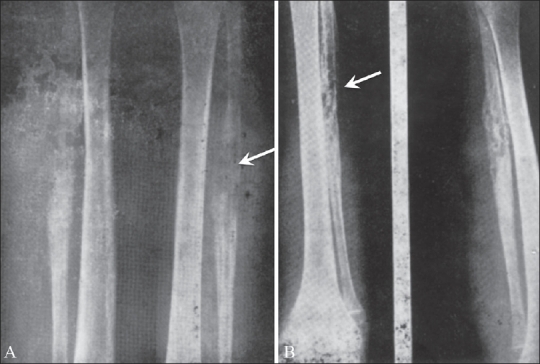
Ewing's sarcoma of the fibula. Pre-irradiation radiographs (A) show a destructive, osteolytic lesion of the fibular diaphysis (arrow). Post-irradiation (B) radiographs show a mottle appearance with evidence of healing (arrow)
